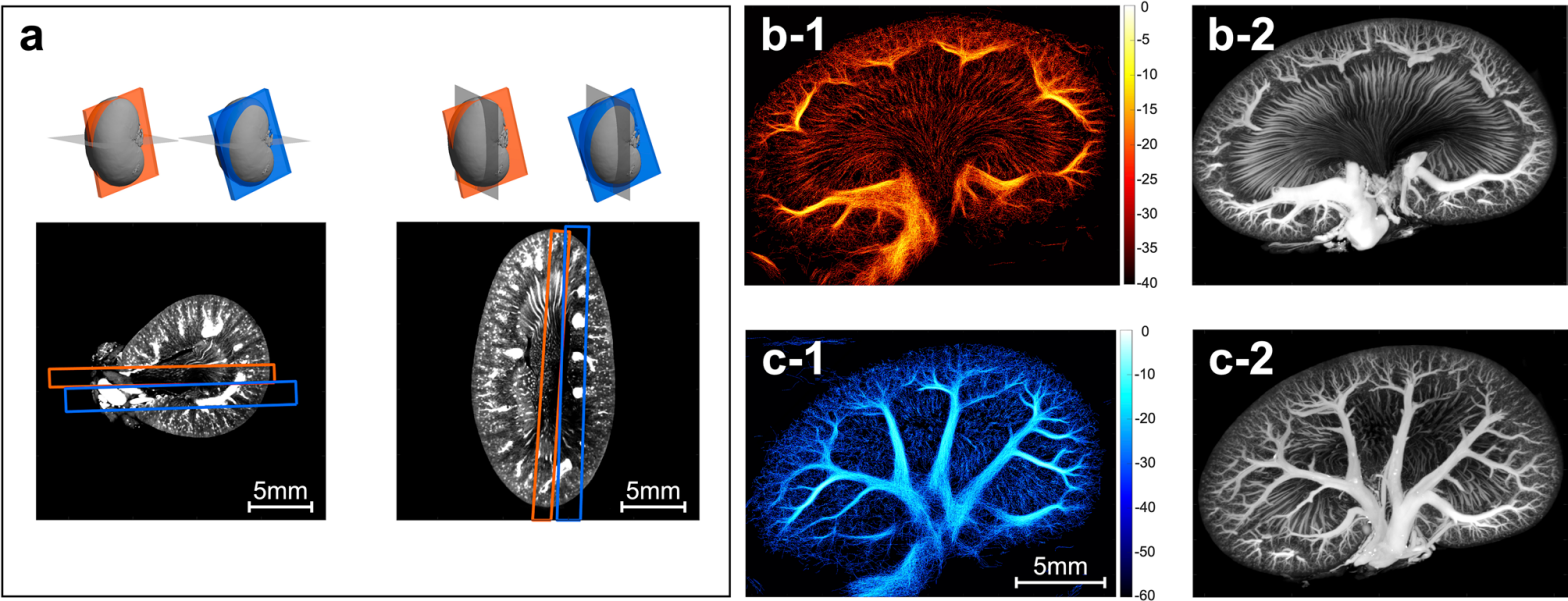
Co-registration of *μ*CT and super-resolution ultrasound images. (a) Co-registration of the two 2D super-resolution ultrasound images to the *μ*CT volume. The images show the two ultrasound fields of view in the *μ*CT coordinate system in an axial *μ*CT slice to the left and a sagittal *μ*CT slice to the right. (b-1,c-1) show the two super-resolution ultrasound images (intensity maps). The images are log-scaled with a dynamic range of 40 and 60 dB, respectively. The color bar shows the value of intensity after logarithmic compression. Intensity corresponds to the number of detected microbubbles. (b-2,c-2) show the *μ*CT maximum intensity projections in the super-resolution ultrasound image overlap.
